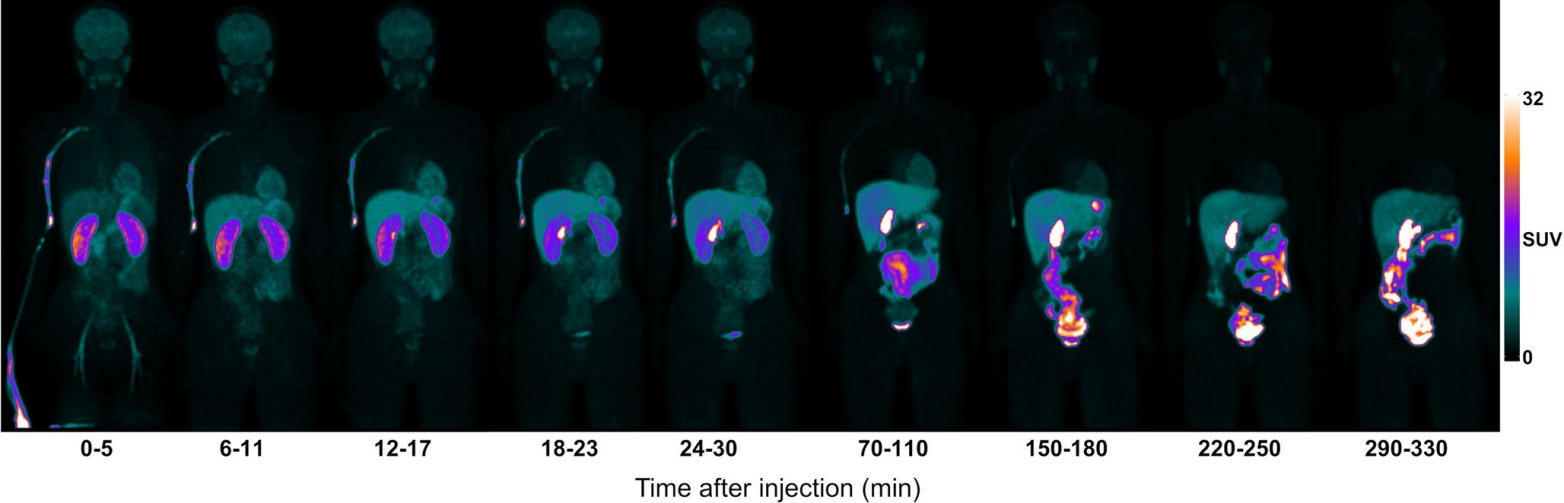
Representative whole-body PET images demonstrating the dynamic biodistribution of [^18^F]SMBT-1 over time. Images are demonstrated in maximum intensity projection (MIP). PET images are demonstrating the dynamic whole-body biodistribution of [^18^F]SMBT-1 to various organs from the early (first 5 min p.i.) to late phases (290 to 330 min p.i.) in a single subject. Early-phase images showed rapid physiological tracer uptake in the kidneys, liver, stomach, heart, spleen, brain, submandibular glands, and parotid glands. Late-phase images showed increased accumulation in the gallbladder, intestine, and urinary bladder. The color scale indicates SUV, ranging from 0 to 32. Abbreviations: MIP, maximum intensity projection; PET, positron emission tomography; SUV, standardized uptake value
